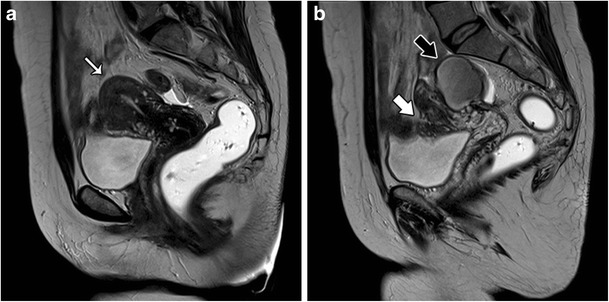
Adenomyosis and endometriosis: a and b Sagittal T2-weighted images; broadened junctional zone forming an ill-defined area of low signal intensity, with punctate high-intensity myometrial foci indicating adenomyosis (thin white arrow); endometriotic nodule in the bladder wall (white arrow); endometrioma in the left ovary (black arrow)
